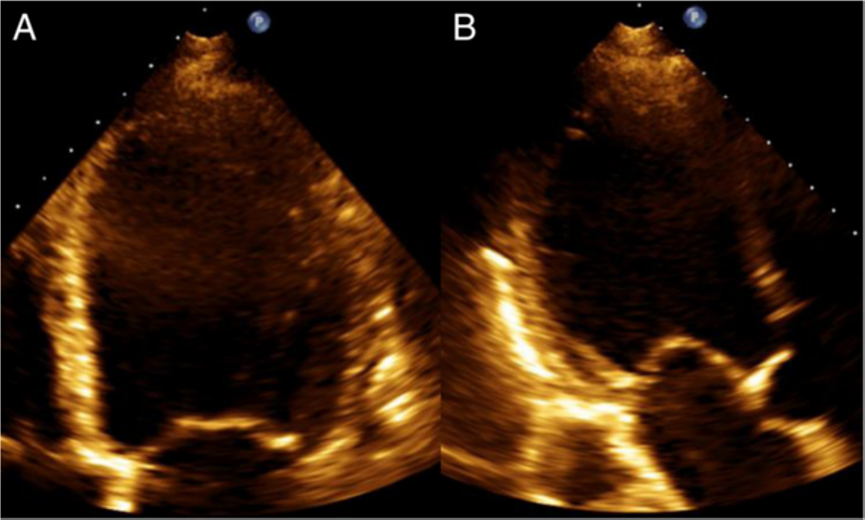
Symmetric tenting due to ischemic LV dilatation. These TTE images were obtained from a 72 year-old male with severe multivessel coronary disease and an advanced ischemic cardiomyopathy with LV ejection fraction of 14% and an LV end-diastolic dimension of 71 mm prior to coronary bypass surgery. A phenotype of symmetric tethering is depicted by these mid-systole images obtained from the apical three chamber view (A) and apical four chamber view (B). The parasternal long axis view is shown as panel A of Figure 4. Measured in the parasternal long axis image, the tenting height was 1.4 cm, the tenting area was 4.0 cm^2^ and the tethering angles β and α were equal. MR severity was graded as trace. Compared to the patient described in Figure 3, the same tenting height and tenting area were associated with markedly distinct CIMR severity, reiterating that tenting phenotype is of utmost importance in determining severity.
